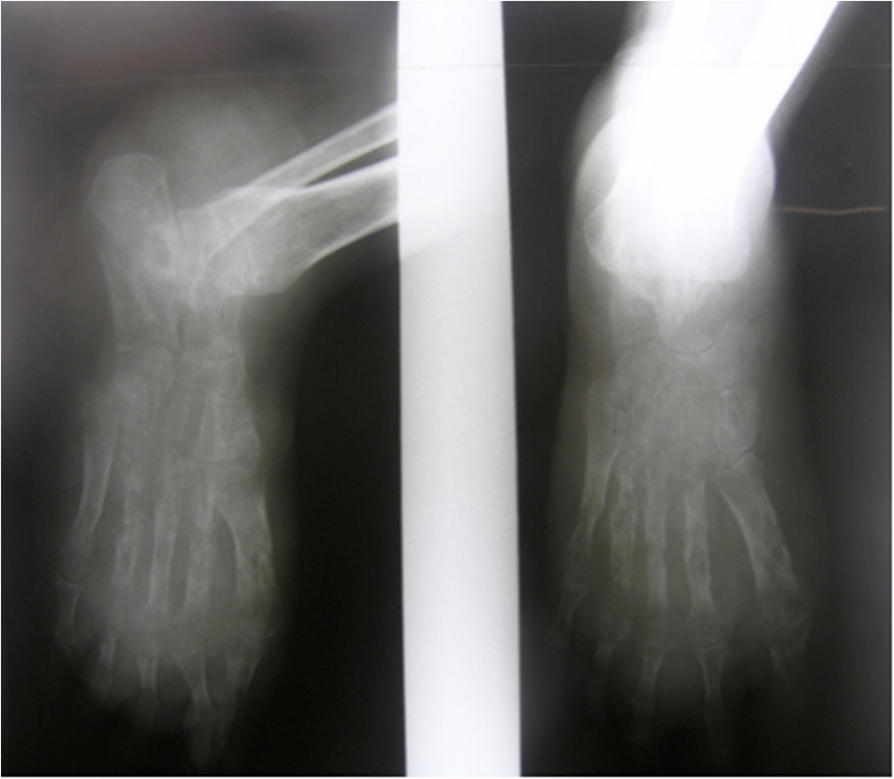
X-ray of the left foot showing sign of destruction of the metatarsal bones.
